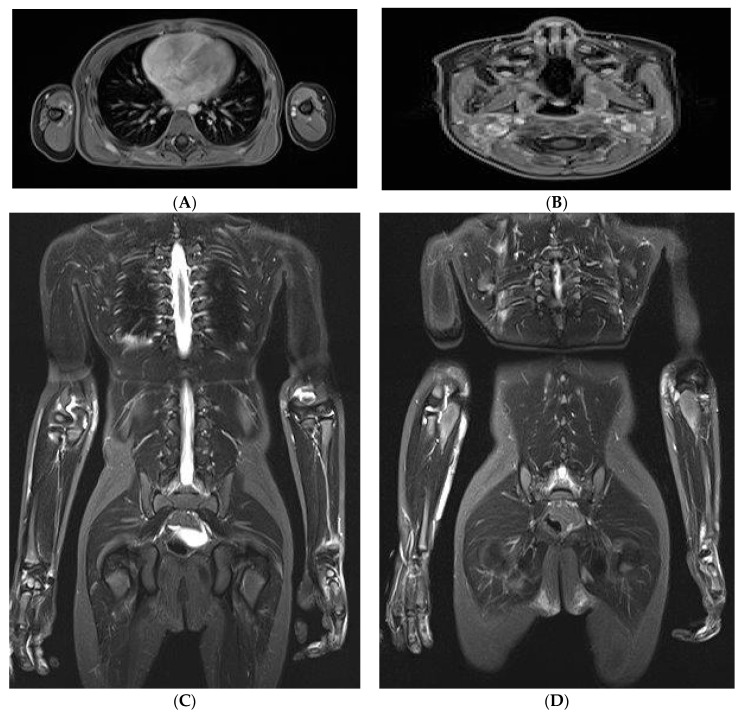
Whole-body MRI imaging: multiple muscular edemas and contrast enhancement consistent with myositis, adjacent to the scapula (A), cervical region (B), arthritis of elbow, hand (C), and multiple cutaneous and subcutaneous edematous and contrast-enhancing inflammatory changes with maximum intensity in the forearms (D). Images courtesy of the Department of Pediatric Radiology, University hospital Tuebingen.
